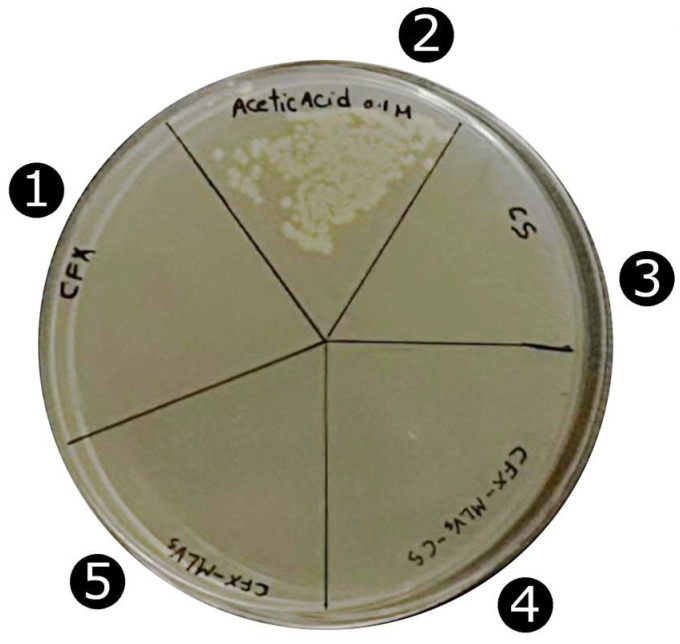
Antibacterial activity of CFX and its formulations. Ciprofloxacin (1), acetic acid 0.1 M (2), chitosan solution 0.3% (3), CFX-SUVs-CS (4), and CFX-SUVs (5).
